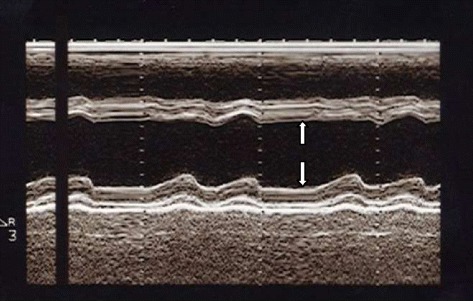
Parasternal long axis view at ventricular level showing left ventricular dilatation (left ventricular end diastolic diameter = 60 mm, white arrows).
